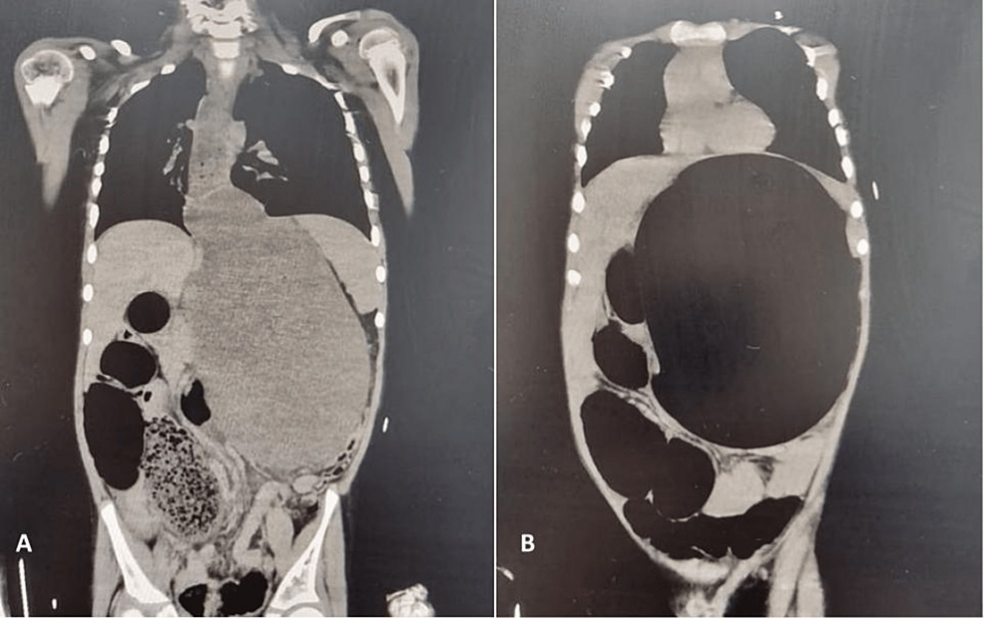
Noncontrast CT abdomen (coronal sections through the abdomen) Significant distention of the stomach and distal esophagus can be seen. Air is also seen in the small bowel CT: computed tomography
